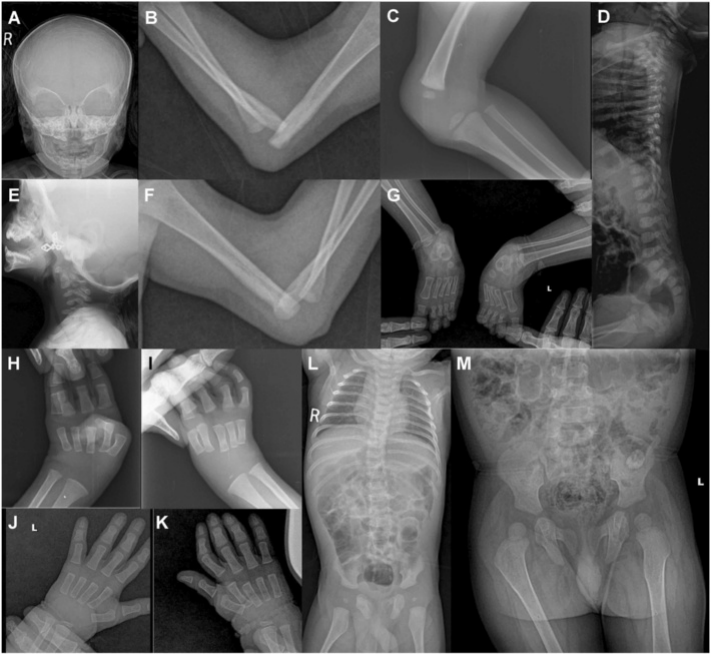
Radiographs of patient 2. He had normal skull (a), bilateral elbow dislocation (b and f), absent patella and dislocated knee (c), coronal clefts in vertebrae (d), cervical kyphosis with subluxation at C4-C5 (e), double calcaneum ossification with club feet (g), delayed carpal ossification at 3 months (h and i), and 1 year (j and k), thoracolumbar scoliosis and small capital femoral epiphysis at 3 months (l), and at 1 year (m)
